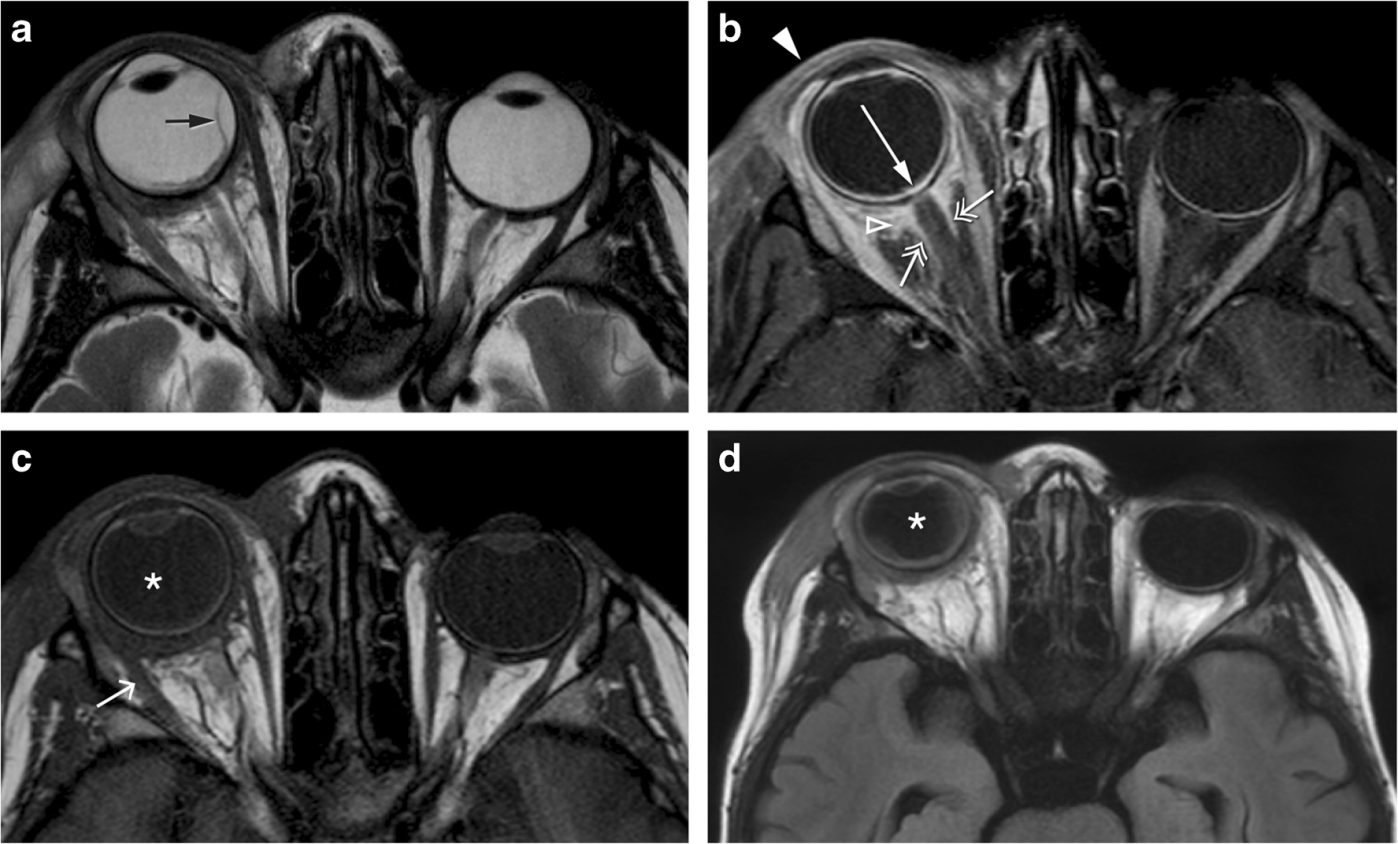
8-year-old male with graft versus host disease after stem cell transplant. Extensive orbital cellulitis, myositis involving the lateral rectus, and sclerouveitis on the right. Axial T2-WI (a), axial T1-WI (c), enhanced axial T1-WI with fat signal suppression (b), and axial FLAIR (d): increased thickness and enhancement of the whole uvea consistent with uveitis (long arrow), subretinal effusions (black arrow), and slightly increased signal intensity of the vitreous humor signal on T1 and FLAIR consistent with vitritis (asterisk). Extensive periscleral cellulitis due to scleritis (empty arrowhead), preseptal cellulitis (arrowhead), myositis of the lateral rectus (short arrow) and perioptic neuritis (double headed arrows)
